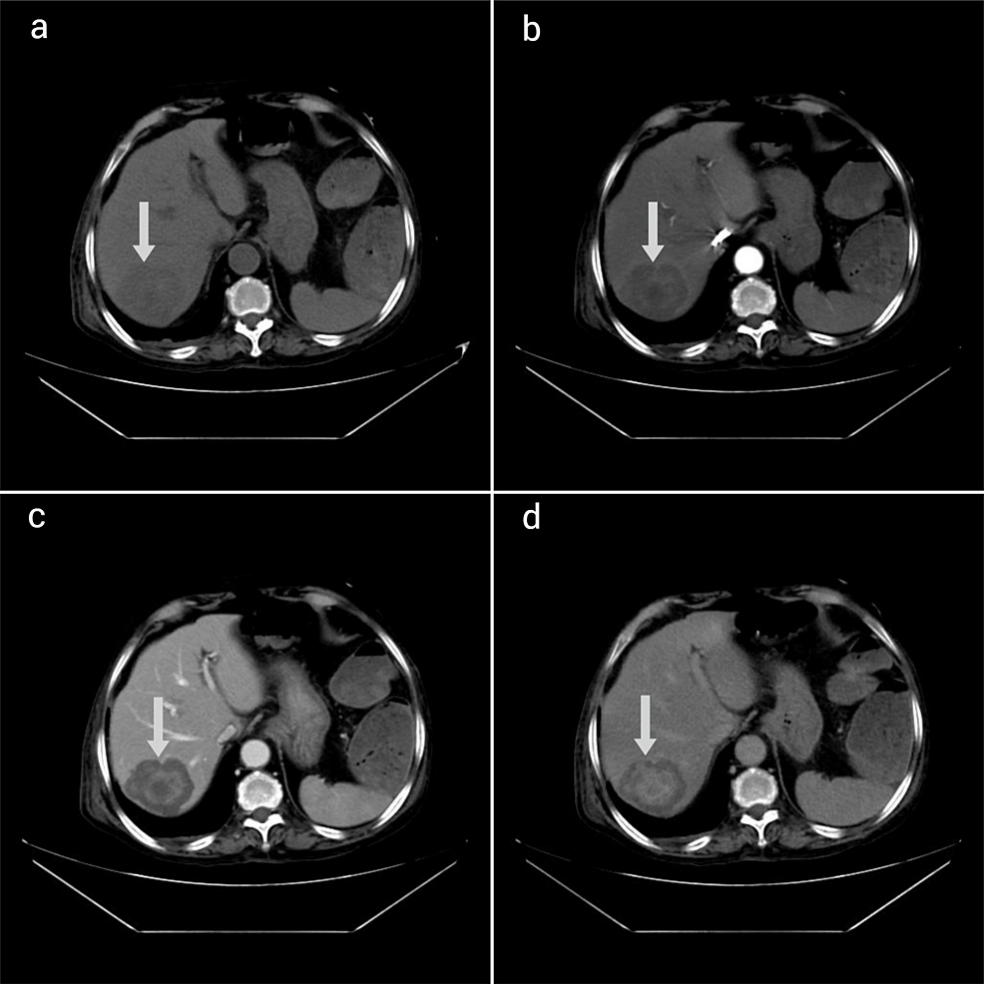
Axial image of non-contrast CT of the upper abdomen (a) shows a well-defined hypodense lesion in segment VI of the liver. On post-contrast axial images, the lesion shows central arterial phase hyper-enhancing areas (b) with progressive centrifugal enhancement in portal venous (c) and delayed phase (d) images.
